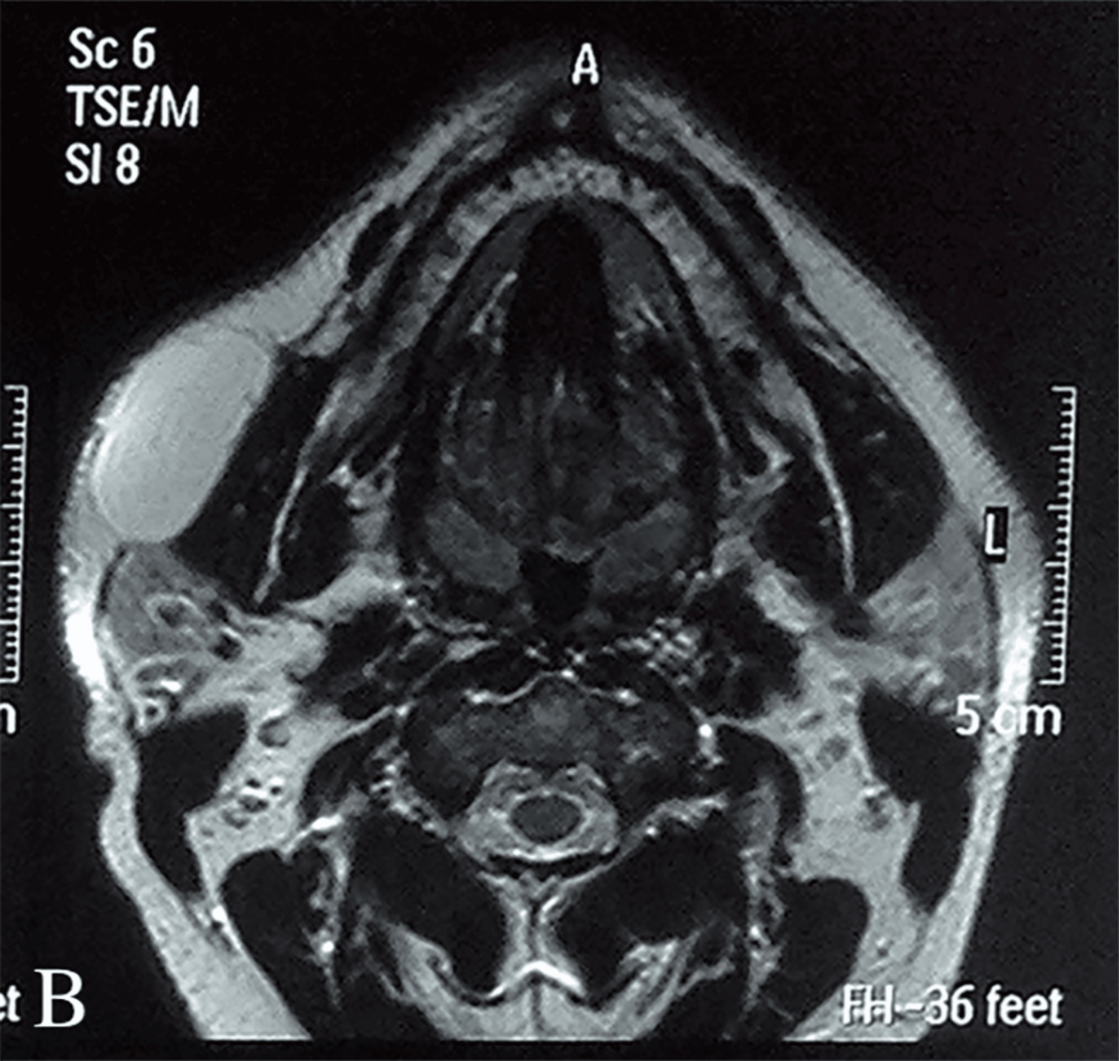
MRI scan revealed a large fatty mass laterally of the buccinator muscle
